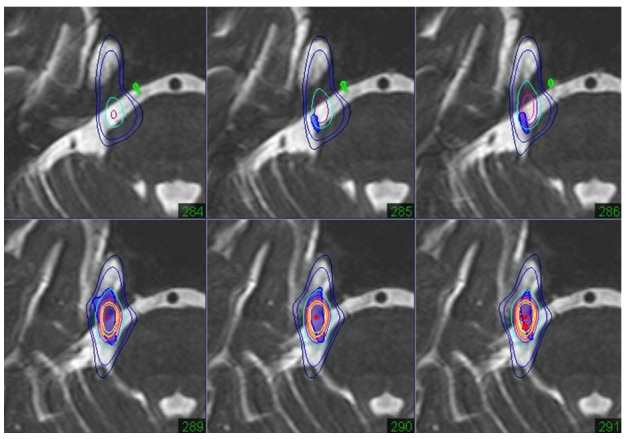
Trigeminal neuralgia. Heavy T2-images (fused to thin-sliced CT) of the trigeminal nerve showing the three branches of the pre-ganglion portion, the Gasserian ganglion, and the retro-ganglion portion. The retro-ganglion portion at the petrous apex is targeted, and the abducens nerve (green) is out of prescribed isodose range.
